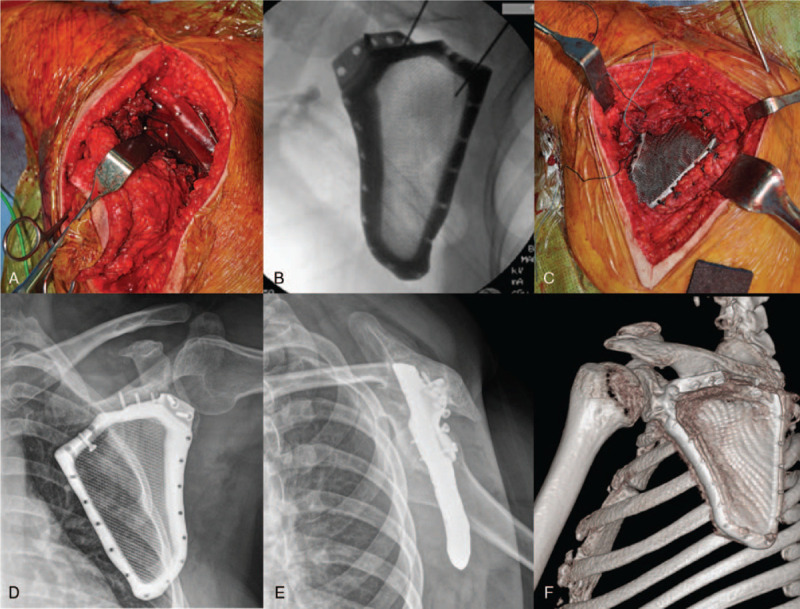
The osteotomy guide to the spine of the scapula and removal of the infrascapular area (A). After implant fixation to the retained scapula by using intraoperative fluoroscopy (B), the surrounding muscle tissue is sutured to the prosthesis through the holes at the edge of the prosthesis (C). The postoperative plain radiographs (D and E) and computed tomography images (F) show good match between the printed segmental scapula prosthesis and the retained scapula.
